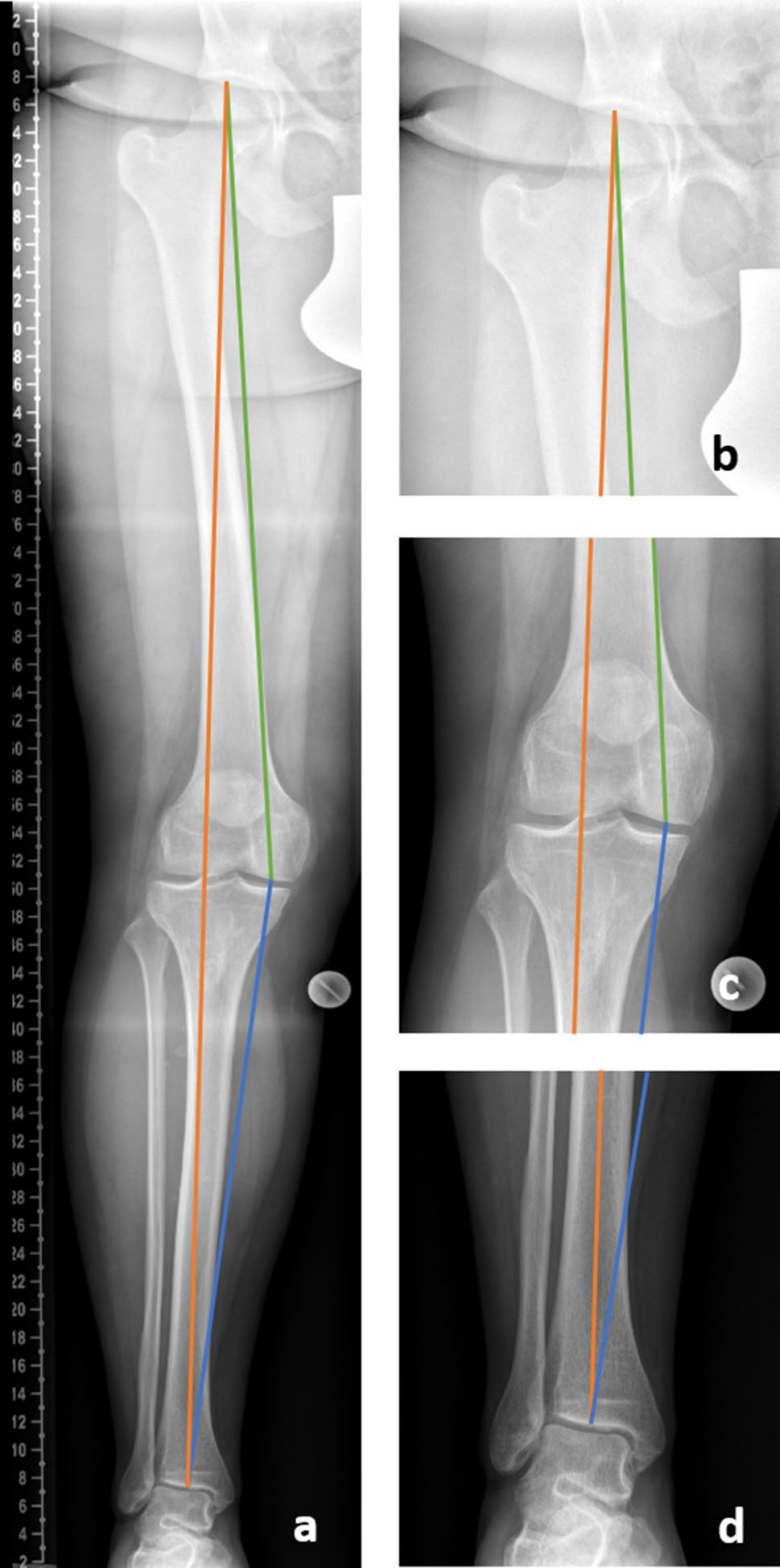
Biometric linear distance measurements taken on the long leg radiographs. Leg length (orange line): distance between the top of the femoral head and the midpoint of the tibial plafond; maximum femoral length (green line): distance between the top of the femoral head and the distal portion of the medial femoral condyle; tibial length (blue line): distance between the distal portion of the medial femoral condyle and the midpoint of the tibial plafond.
